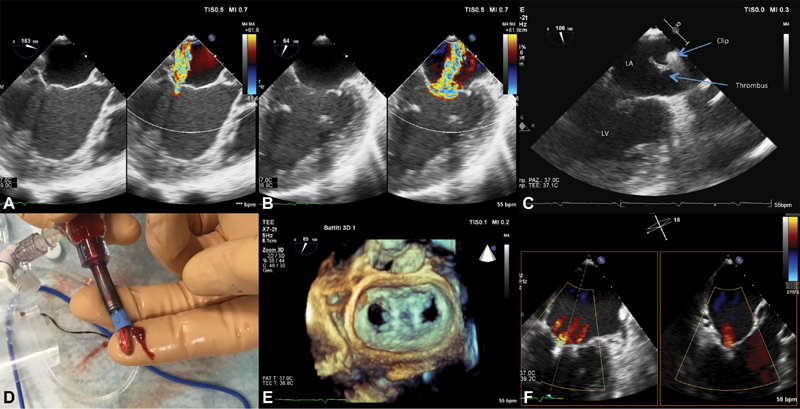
Intraprocedural echocardiography and clip thrombosis. (
